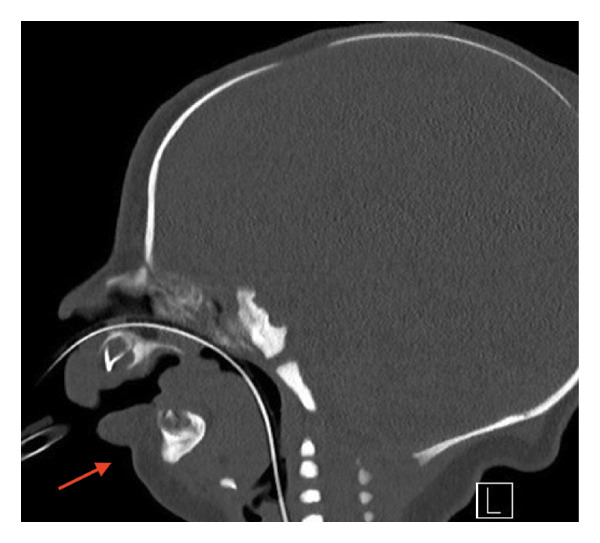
CT scan of facial bones showing significant micrognathia with bilateral hypoplasia of the mandible and mild glossoptosis with narrowing of the posterior oropharyngeal airway and no evidence of bony cleft palate. The micrognathia and mandibular hypoplasia are indicated with an arrow.
